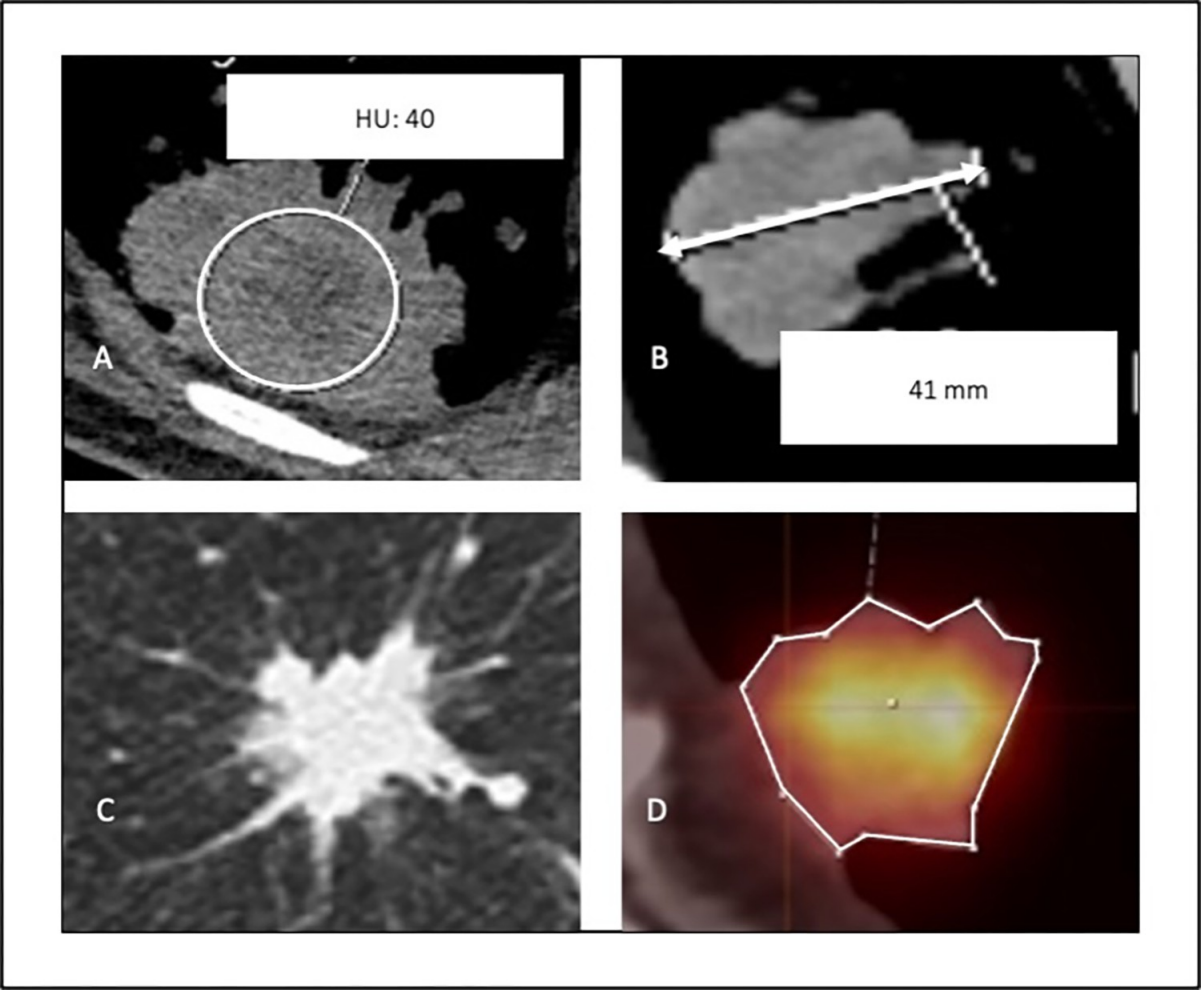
Example of image analysis. (A) calculation of density using a region of interest (ROI), (B) measuring of maximal diameters in mm, (C) evaluation of spicules and (D) measuring the SUV_max_ values in the tumor area with the highest metabolism using a volume of interest (VOI).
